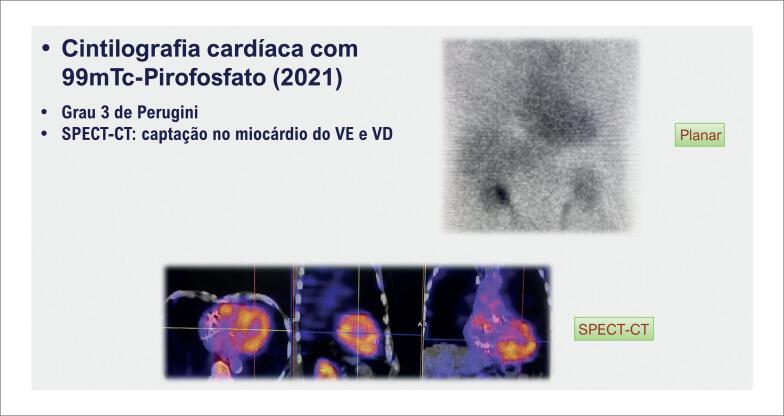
Cintilografia com pirofosfato de 99m-tecnécio demonstrando captação mais intensa do que a das costelas (imagem planar com Grau 3 de Perugini) e confirmada a captação no miocárdio em imagem tomográfica (SPECT-CT). O paciente teve a exclusão de gamopatia monoclonal realizada, sendo confirmado o diagnóstico de amiloidose por transtirretina. SPECT-CT: tomografia computadorizada por emissão de fóton único-tomografia computadorizada (Single photon emission computed tomography-computed tomography); VD: ventrículo direito; VE: ventrículo esquerdo.
